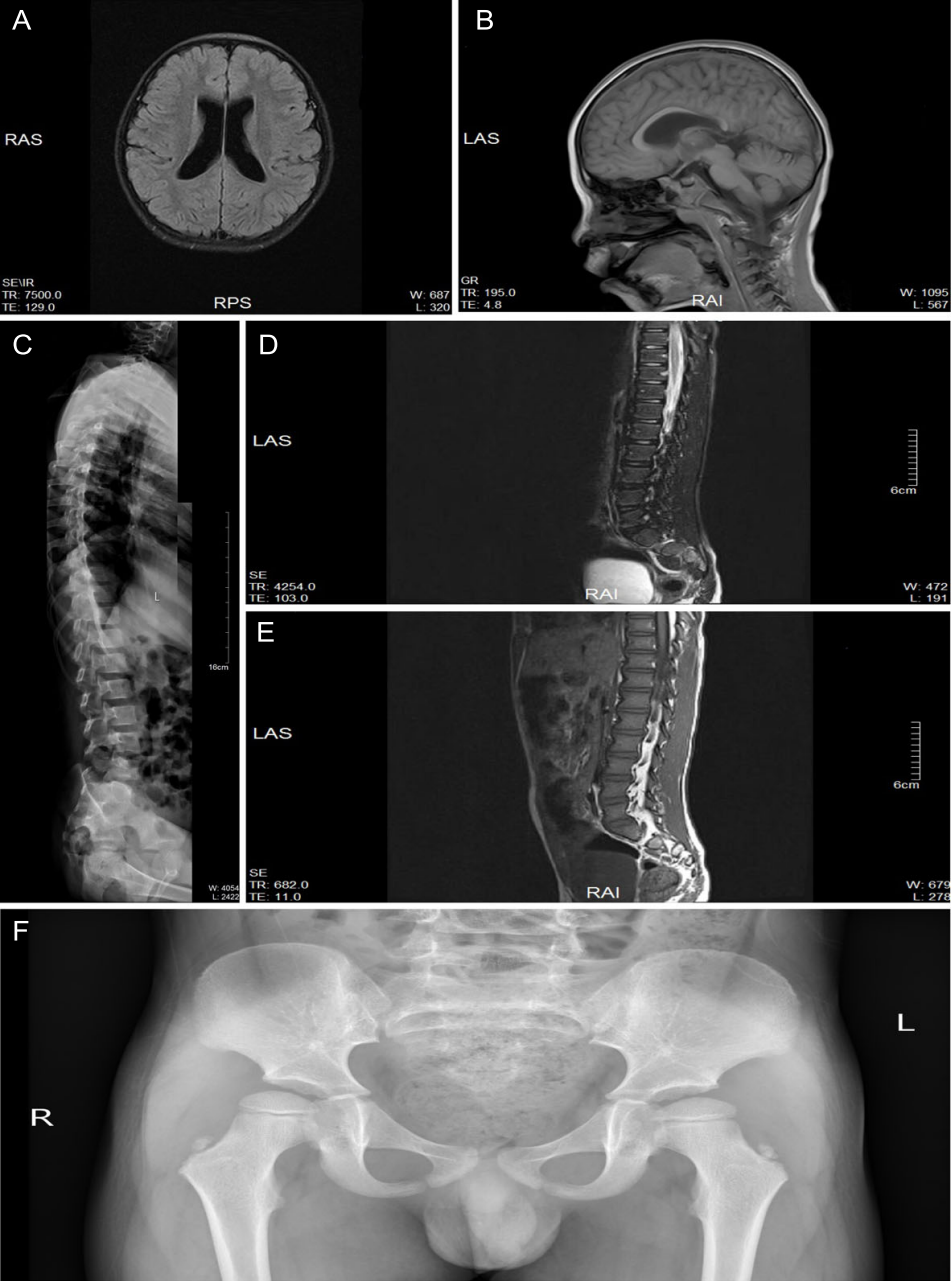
MRI scan results of patient presented unstable walk with lisping at seven-year-old and was referred to a pediatric neurologist at the 7-year-old (201702). a Brain MRIs showed dilated supratentorial ventricle. b Thin posterior part and splenium of corpus callosum. c Total spine MR imaging suggested 1, L4, 5 recessive spina bifida. d Cervical and thoracic spinal cord scan was normal. e Lumbosacral segment of spinal cord scan was normal. f Pelvic radiograph was normal
